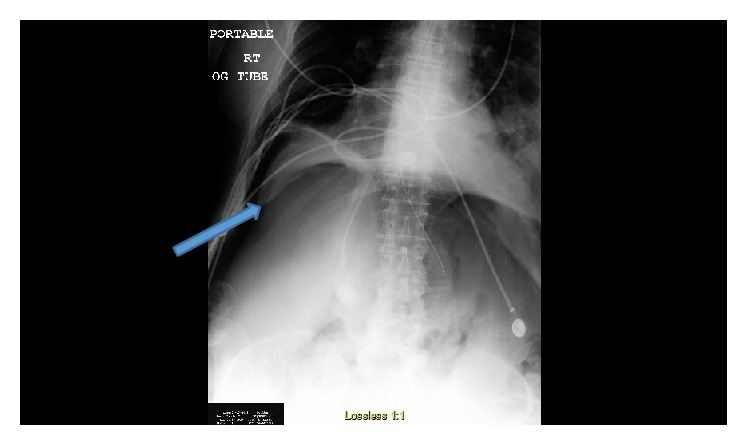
Chest/abdomen X-ray showing right pneumothorax and also air under diaphragm suggesting perforated viscus.
